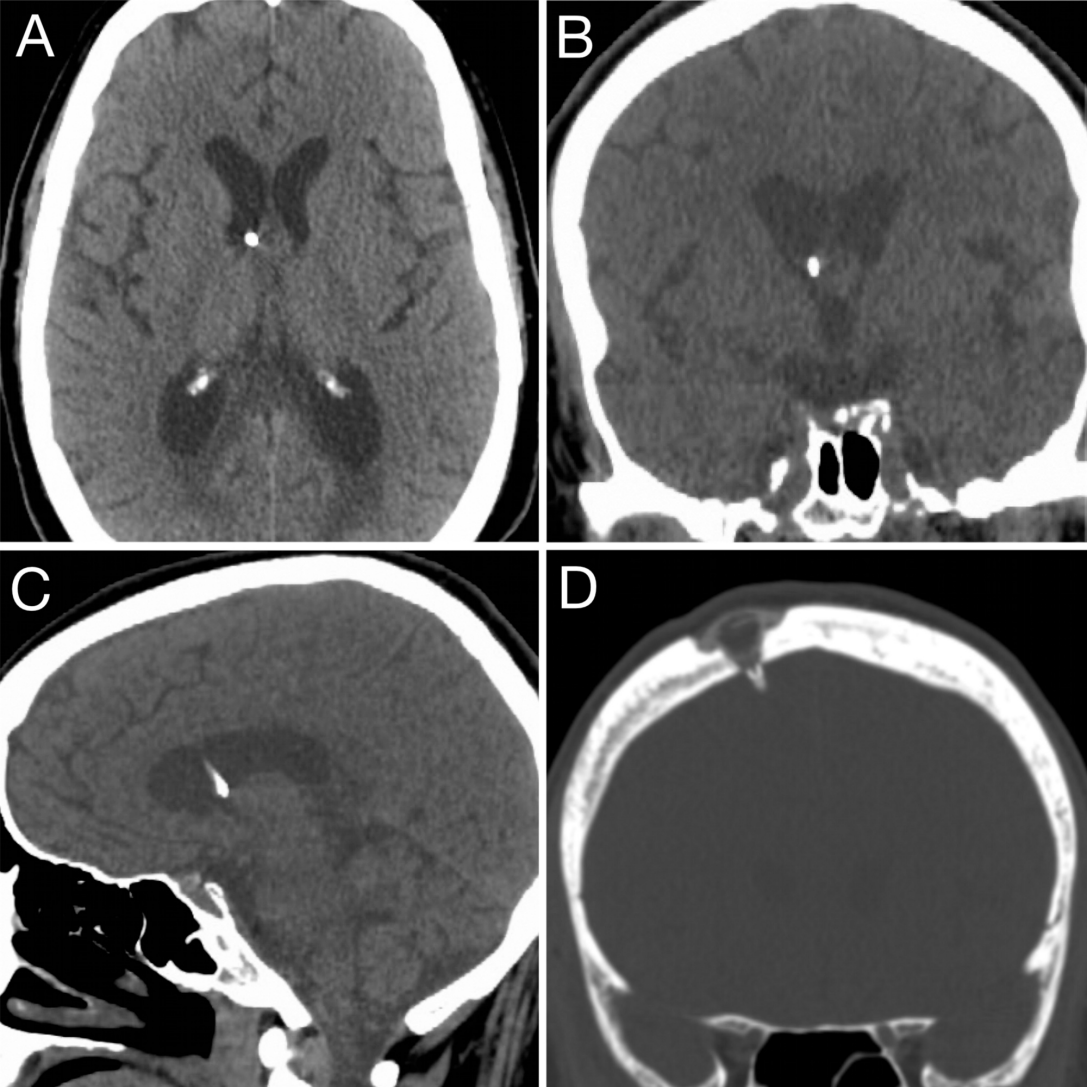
Postoperative imaging. Postoperative noncontrast CT scan showing ideal catheter placement in (A) axial, (B) coronal and (C) sagittal planes. (D) Bone window coronal CT scan showing the recessed Ommaya reservoir and smooth surface of the skin over the reservoir.
